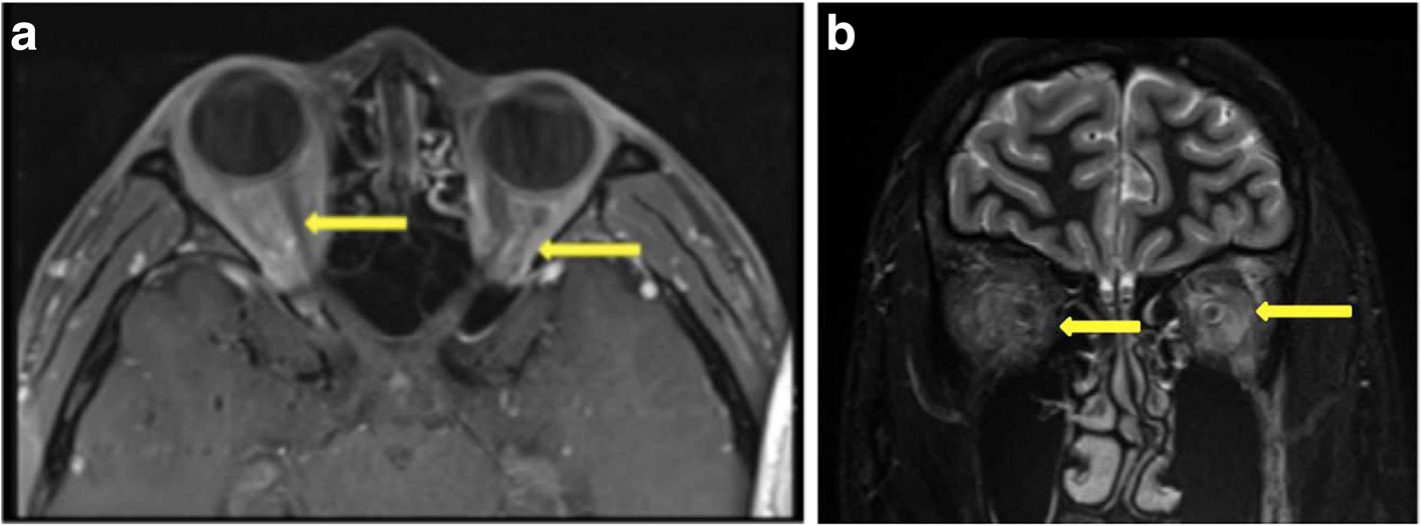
Orbitographic magnetic resonance imaging at recurrence. Right exophthalmos (a) and orbital softtissue inflammation (b) are evident
